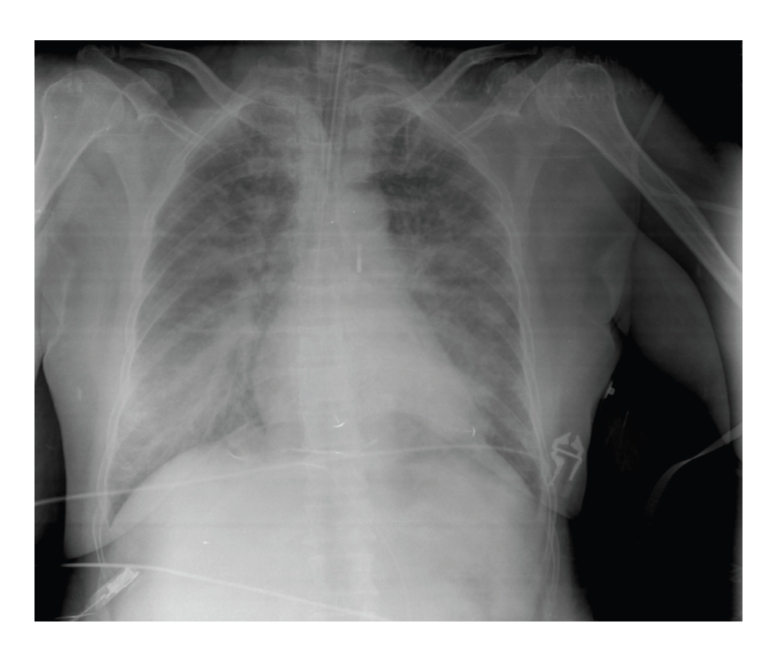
Chest X-ray after Angio-Seal placement.
